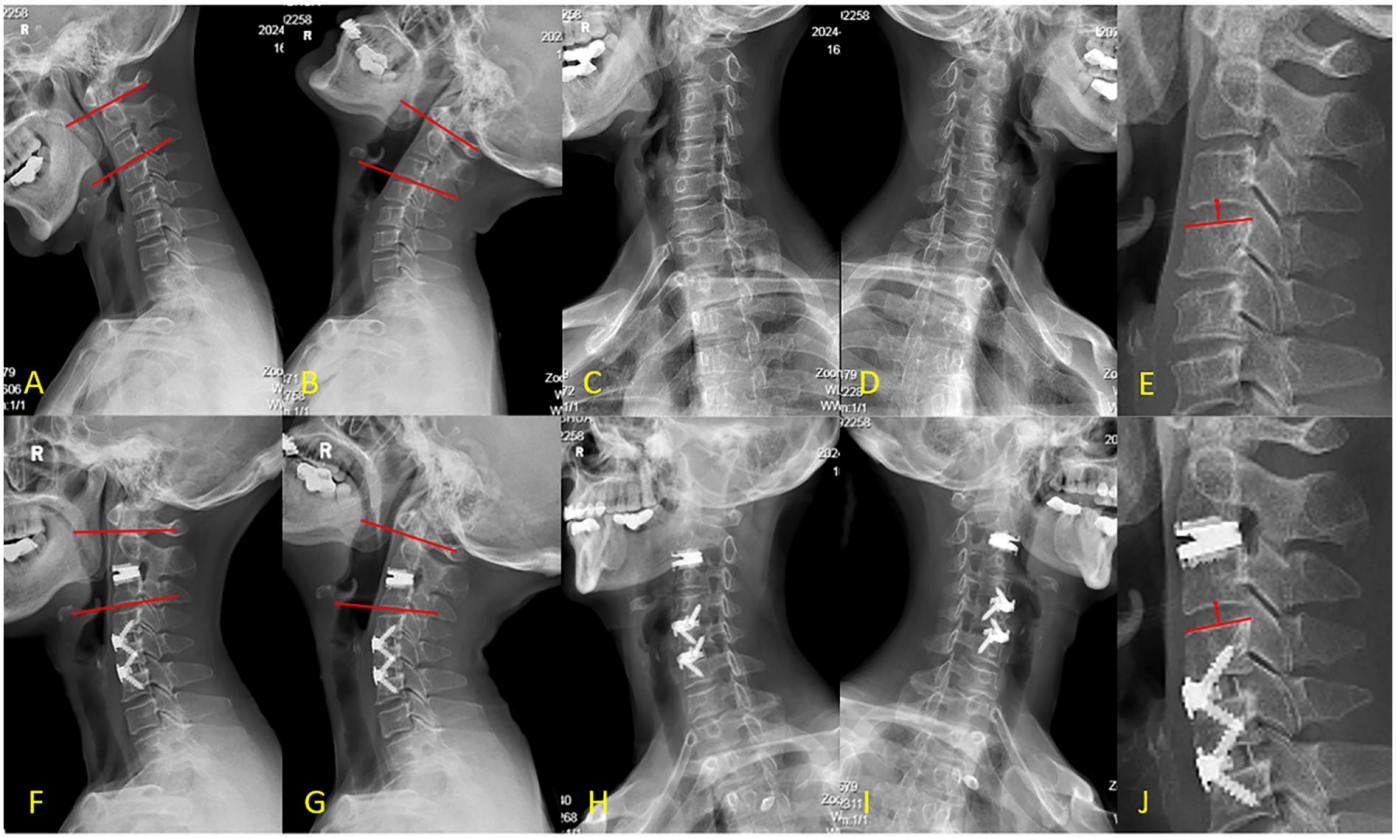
(A–E) Preoperative x-rays, F-J: postoperative x-rays. Image (A) and (B) shows preoperative C2-3 range of motion was 7.1°. Image (F) and (G) shows postoperative range of motion was 6.9°. Image (C) shows stenosis of the C4-5 and C5-6 intervertebral foramina. Image (H) shows complete resolution of the stenosis with the intervertebral foramina enlarged compared to preoperative status. Image (E) shows preoperative intervertebral disc height (IDH) of C3-4 is 14.1 mm, Image (J) shows postoperative IDH of 13.4 mm with no loss.
